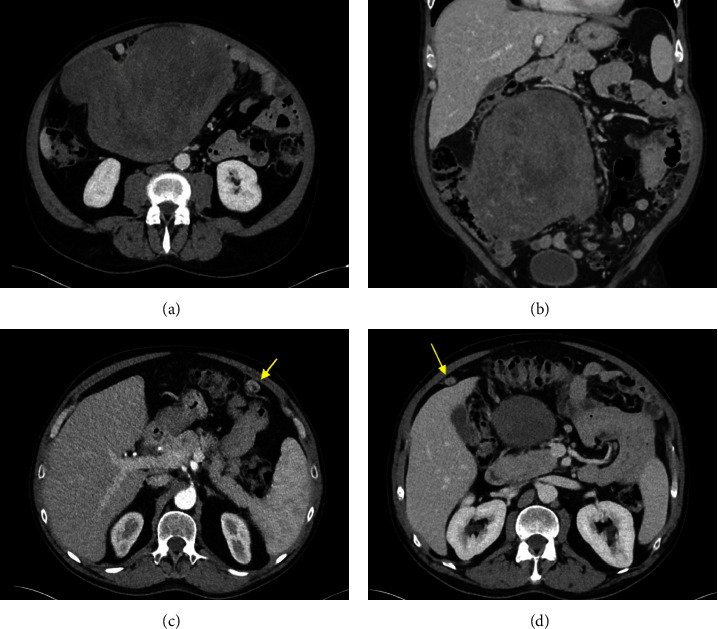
Representative images of the patient's CT abdomen/pelvis with IV contrast showing a large, lobulated heterogeneous abdominal mass in images (a) and (b) and omental nodules in images (c) and (d).
